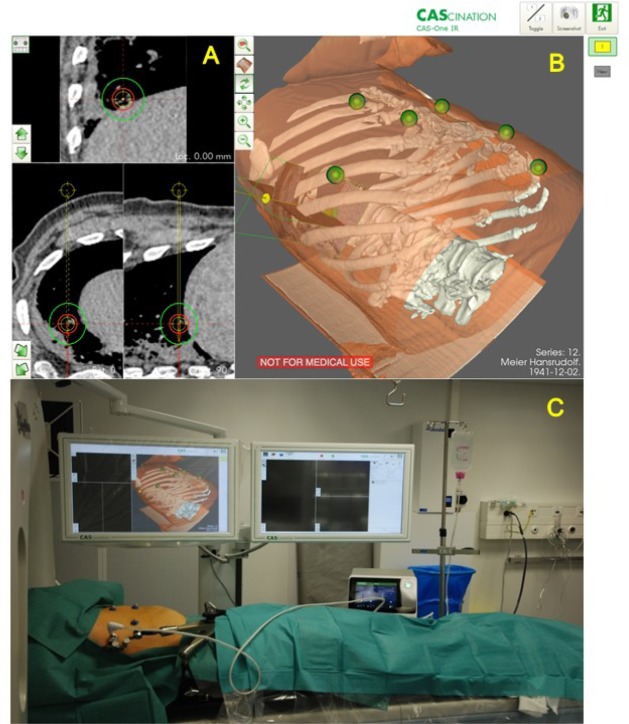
A) Planning of the ablation in the CT scan with targeting of the lung metastasis (red circle) and microwave ablation zone (green circle) B) Planning with the 3D-reconstruction to plan the pathway (yellow) and length of the needle and the six synchronization markers on the patient (green) C) Set-up with the two touch-screen
